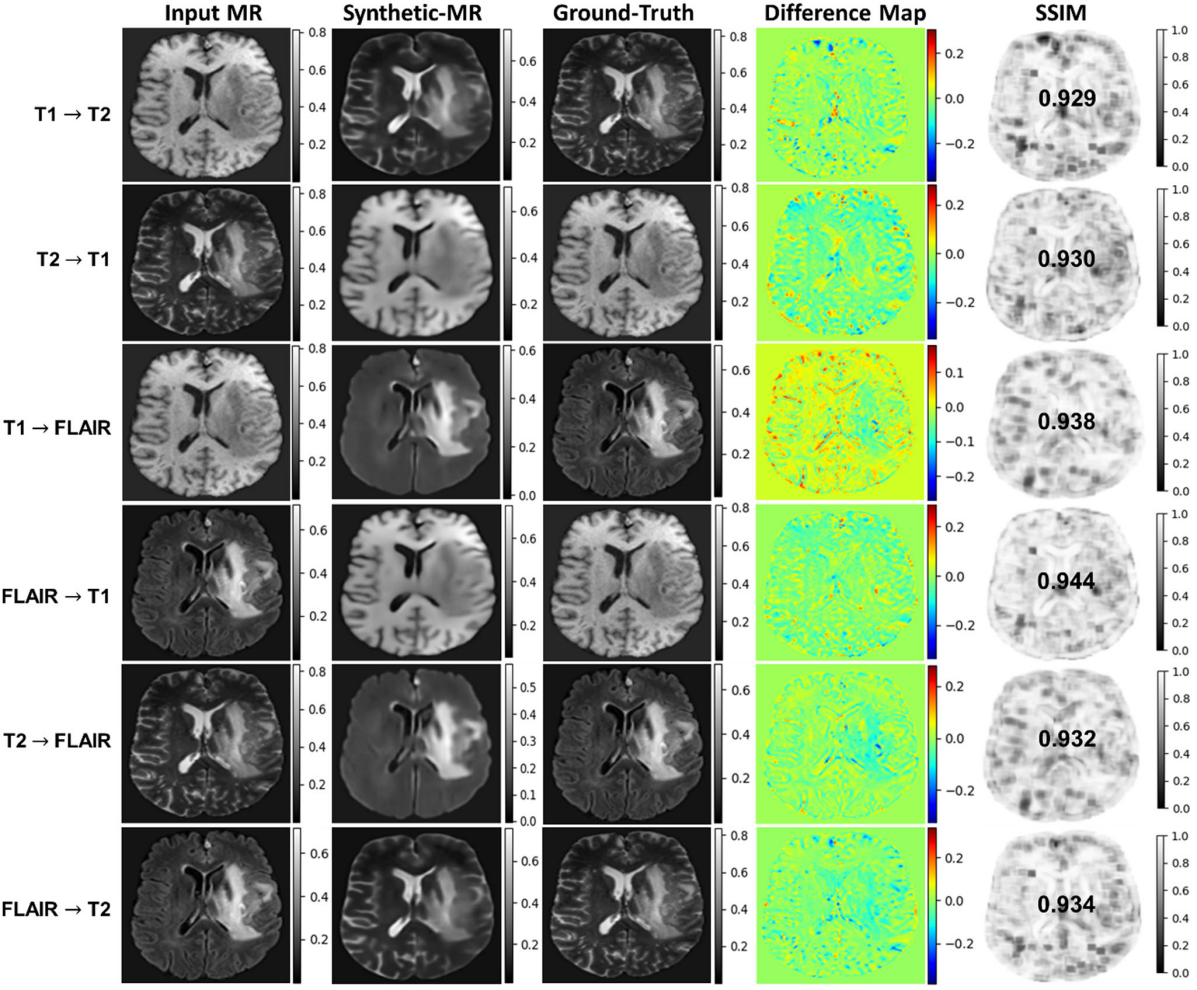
The comparison of synthetic‐MR images generated using a U‐Net model for one subject on a test data set. From left to right: input MR images (source image contrast); synthetic‐MR images (target image contrast); ground‐truth/real MR image (target image contrast); difference map (predicted–real MR image); and SSIM map. Rows show image‐to‐image translations across T1, T2, and FLAIR MR contrasts
